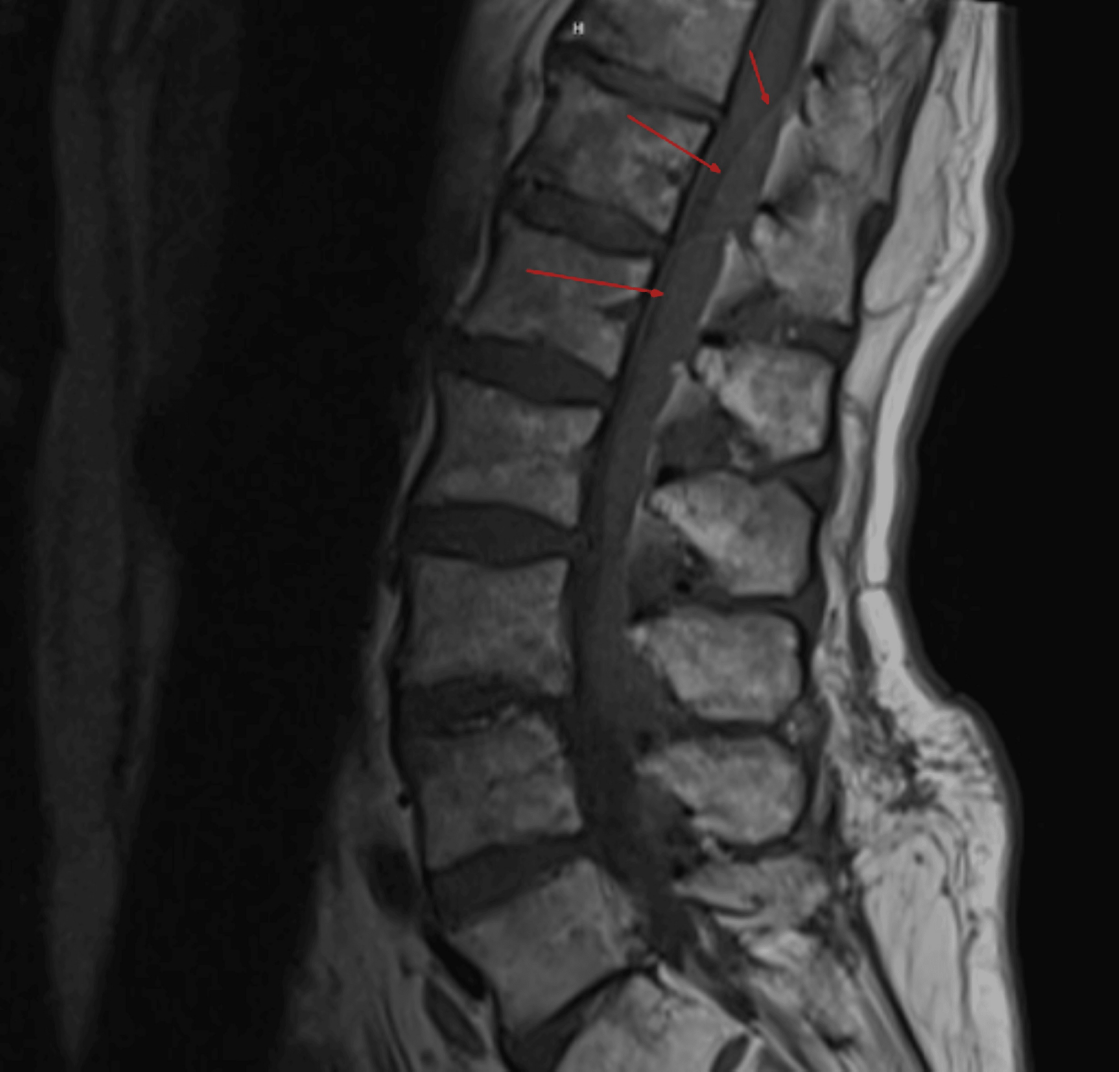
T1 sagittal MRI demonstrates a delineated hyperintensity within the spinal canal displacing the cord anteriorly. MRI: magnetic resonance imaging
